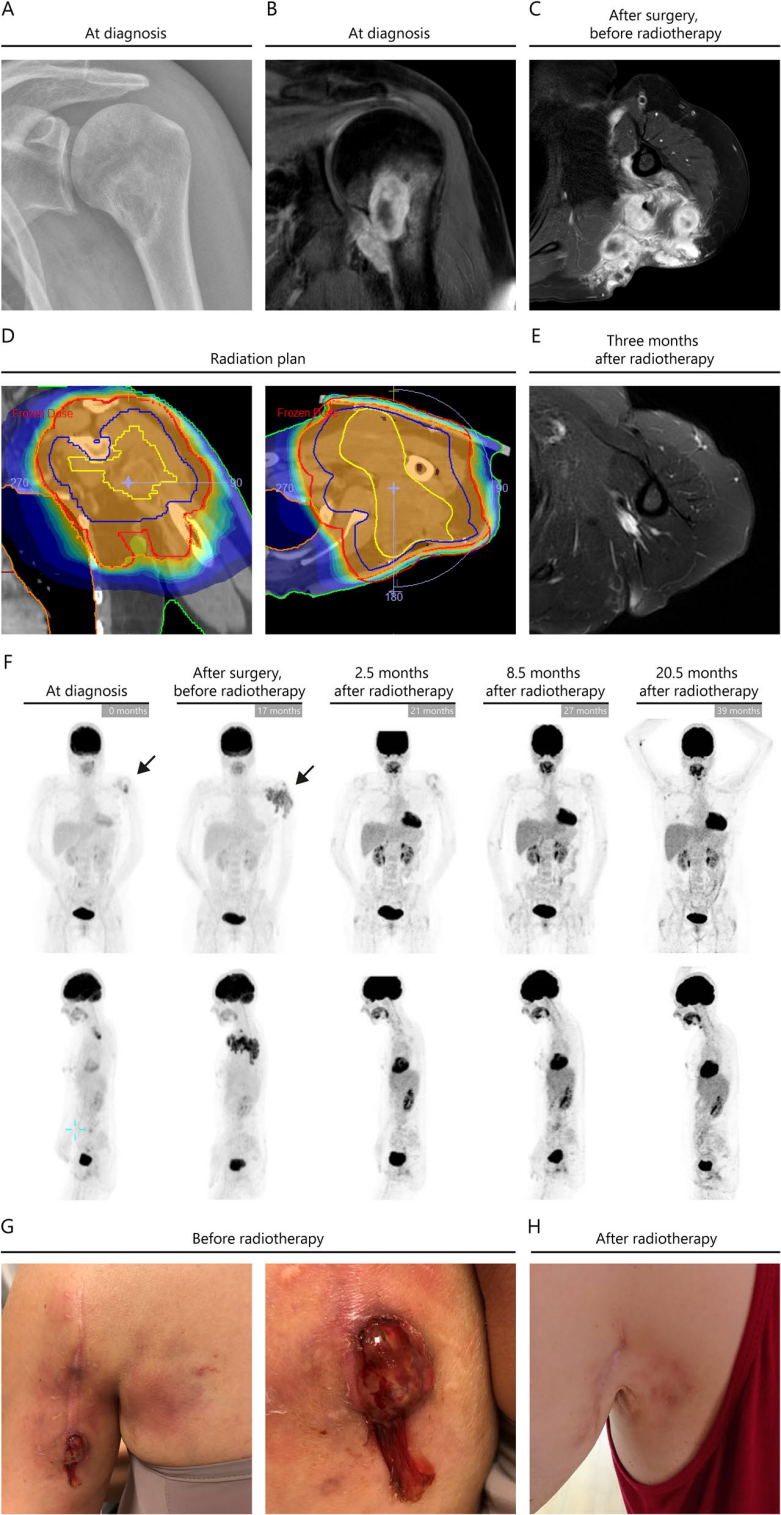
Coronal X-ray (A) and gadolinium-enhanced T1-weighted MRI (B) images demonstrating a unifocal lesion in the left proximal humeral diaphysis at time of diagnosis. After seventeen months and two surgical interventions, axial gadolinium-enhanced T1-weighted MRI demonstrated progressive disease with significant soft tissue extension (C). Radiation treatment plan of the humerus. The planning target volume (PTV) is indicated by the red line, the clinical target volume (CTV) by the blue line, and gross tumor volume (GTV) by the yellow line (D). Three months following RT, T2-weighted MRI demonstrates a full radiological response (E). Successive PET-CT images show strong FDG uptake in the bone and surrounding soft-tissues, with a remarkable decrease in both tumoral mass and FDG uptake after RT, compatible with a complete radiological response (F). Abscess-like soft tissue extension through the skin prior to RT (G). Clinical response at 9 months post RT (20 Gy in 10 fractions) showing marked improvement of skin and subcutis inflammation (H)
